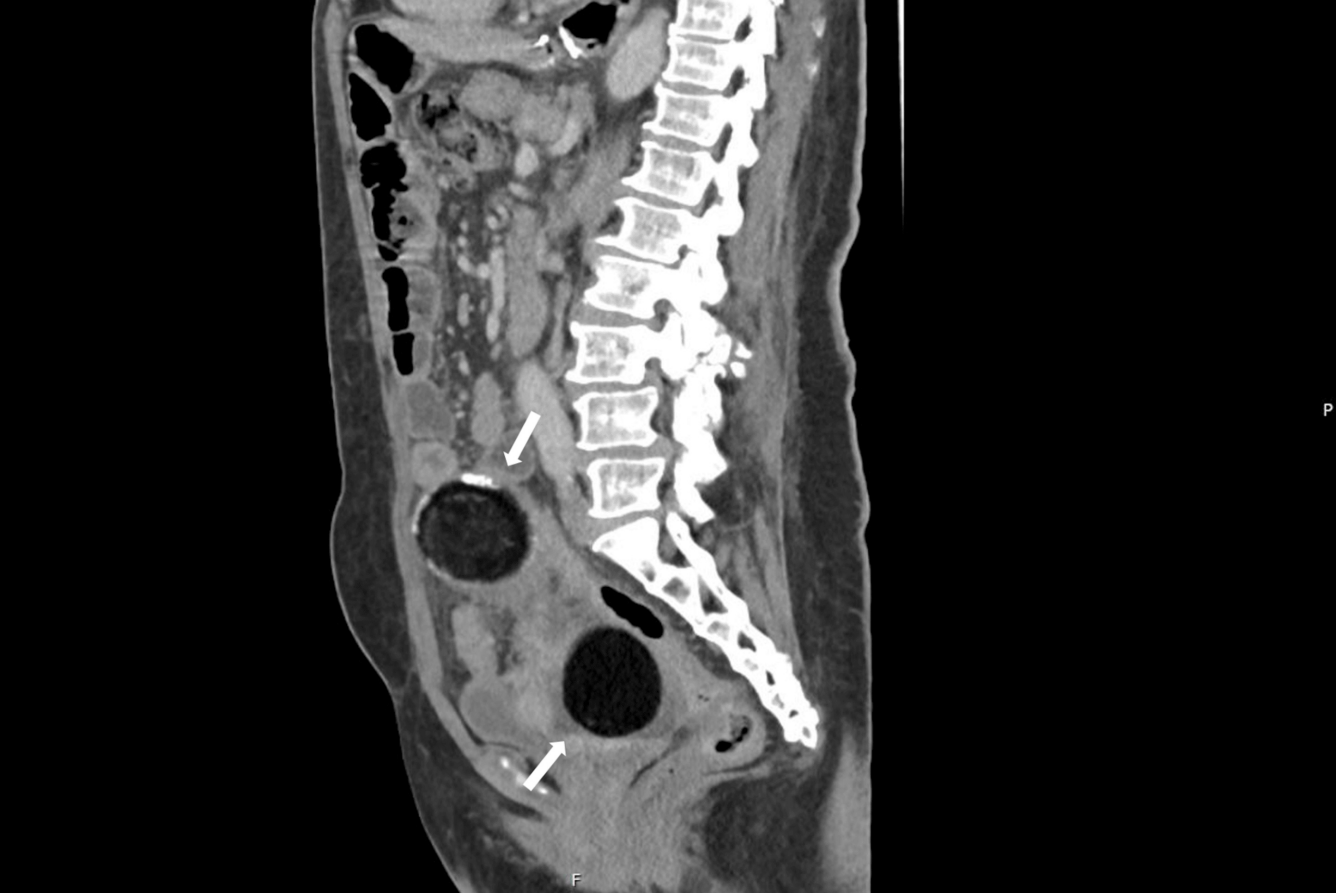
Abdominopelvic CT showing two adnexal masses (arrows), one of them in a much superior location in relation to the pelvis.
